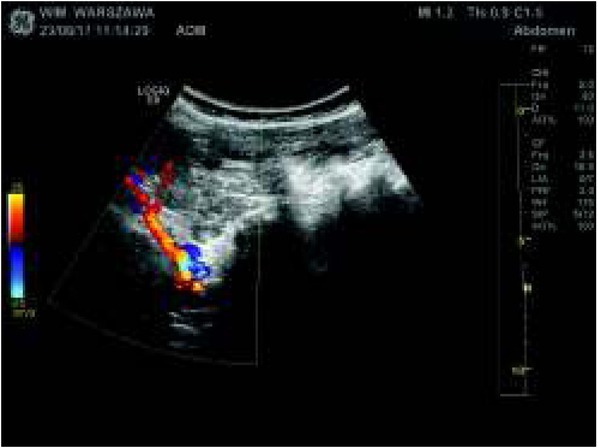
Doppler imaging of renal artery. Ryc. 1. Obrazowanie metodą kolorowego dopplera głównego pnia tętnicy nerkowej.
